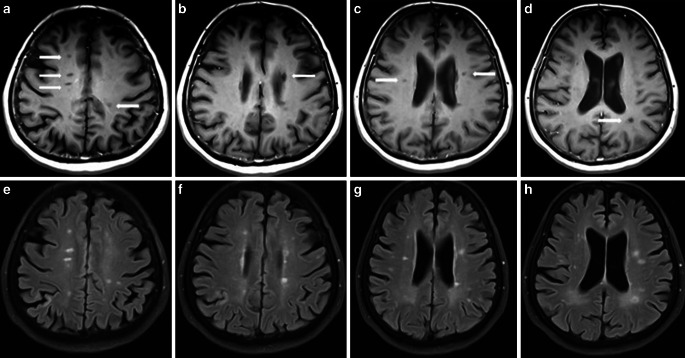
Black holes in four PPMS patients. a–d Axial T1-weighted spin-echo images with hypointense lesions (black holes) in deep and periventricular white matter (*white arrows*), e–h. Axial fluid attenuated inversion recovery (FLAIR) images with corresponding hyperintense lesions in the same locations
